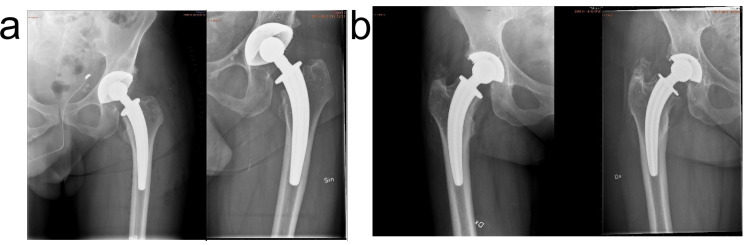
a. Radiography of a stable cup at eight years (right). Direct postoperatively image to the left. b. Radiography of a cup with PE wear at eight years (right.) Direct postoperatively image to the left
